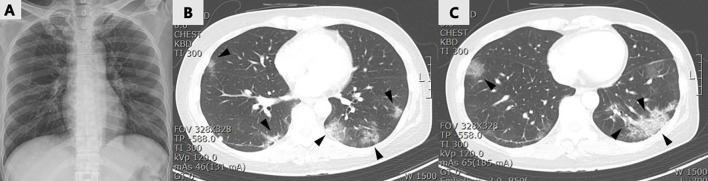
Radiologic findings during the second admission. (A) Chest radiograph showing no definitive active lung lesion. (B, C) Axial chest computed tomography (CT) images showing bilateral patchy consolidations and GGOs with associated interstitial thickening.
